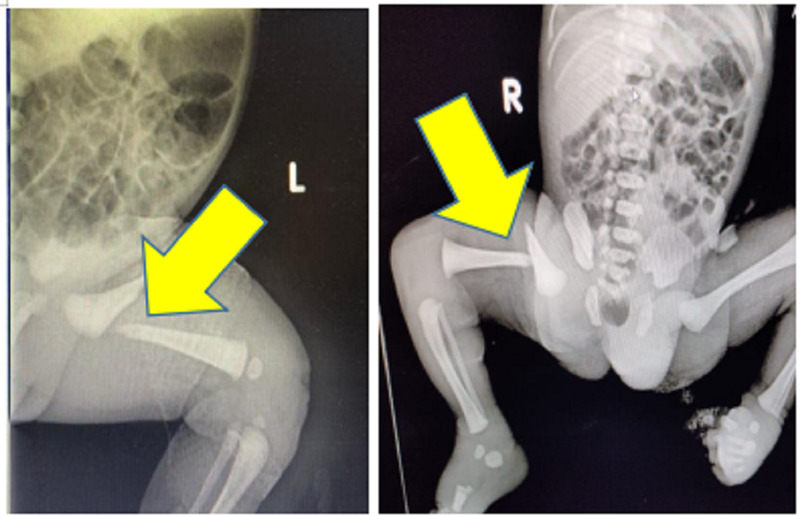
Radiographs of Baby 1 & Baby 2
